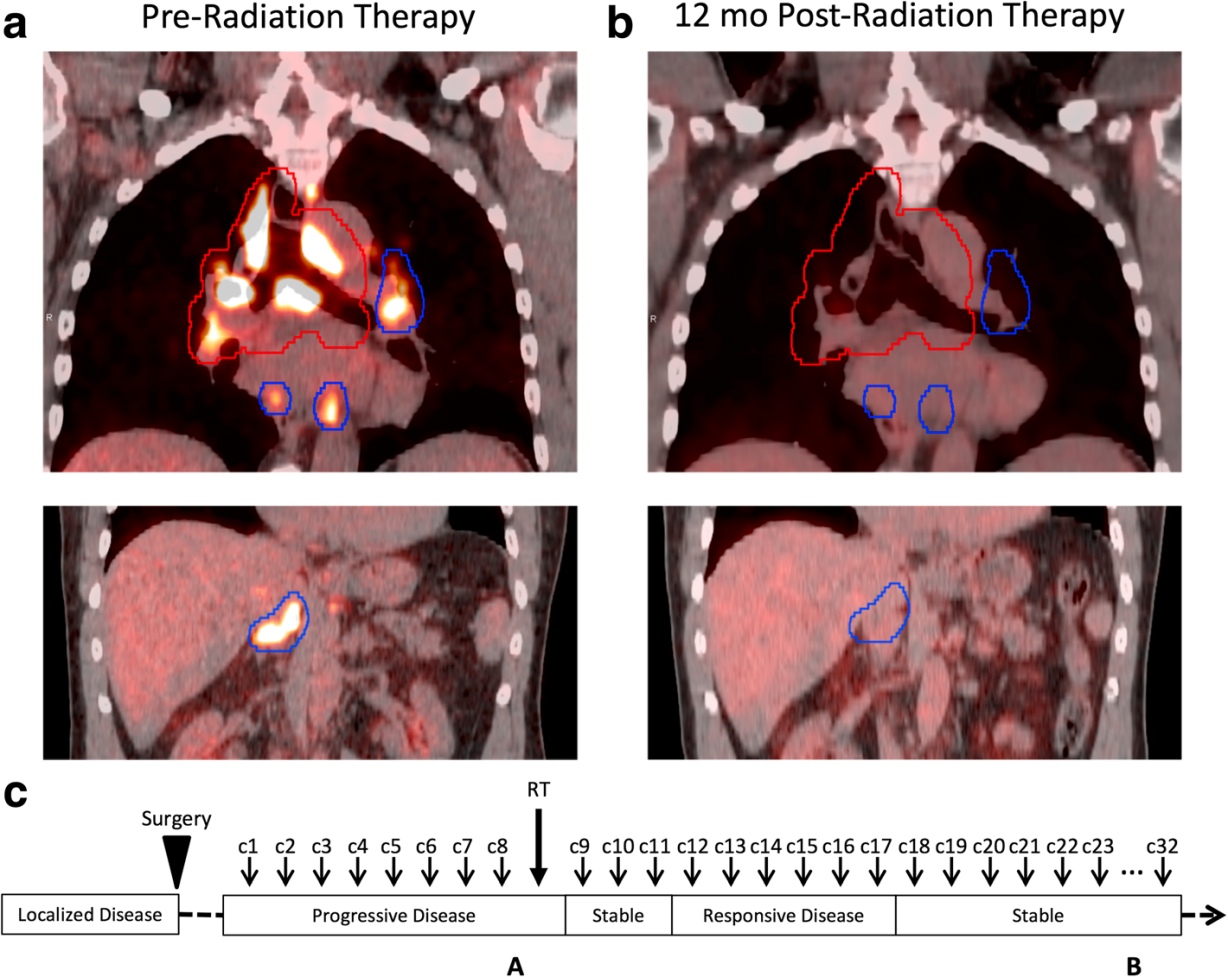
Case B. FDG-avid adenopathy before and after RT with target volume outlined in red and untreated disease outlined in blue. Left (Panel a): Coronal pre-RT PET/CT demonstrating mediastinal FDG-avid adenopathy in the mediastinum and bilateral hila (top) and upper abdomen (bottom); not pictured: supraclavicular adenopathy. Right (Panel b): Coronal PET/CT at 12 months after RT demonstrating radiographic response in the mediastinum and bilateral hila (top) and radiographic response in the upper abdomen (bottom). Panel c: Timeline of therapy and disease status, with A and B corresponding to time points depicted in Panel a and b above. c1 = cycle 1 of pembrolizumab. RT = radiation therapy. PET/CT = positron emission tomography/computed tomography. FDG = fludeoxyglucose
